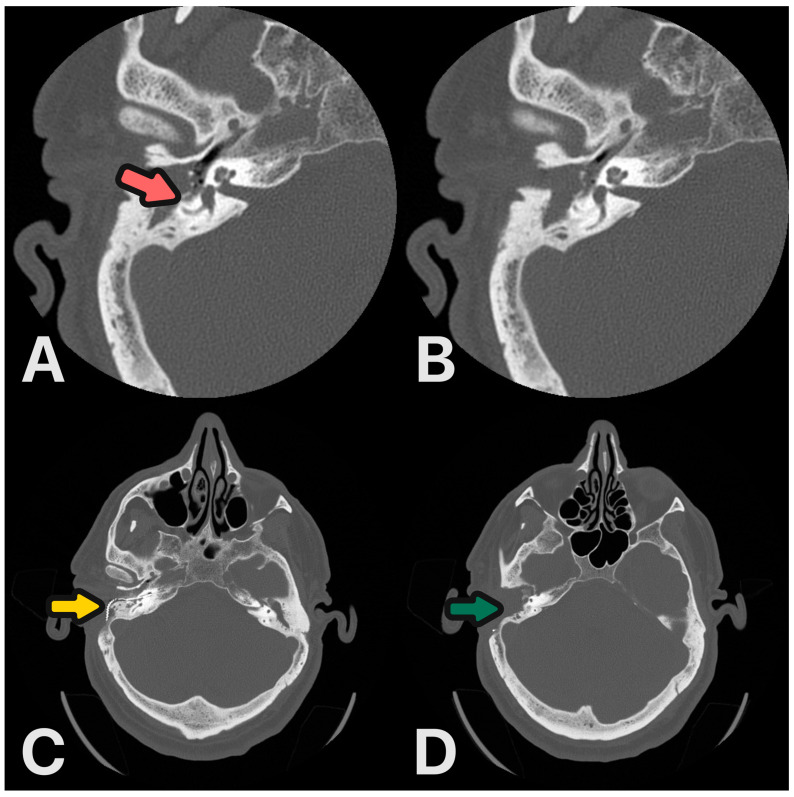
(A,B) HRCT scans before surgery: destruction of the lateral semicircular canal and posterior canal wall—magenta arrow. (C,D) Control HRCT scans 6 months after procedure: CI electrode—yellow arrow, ear obliterated—green arrow.
